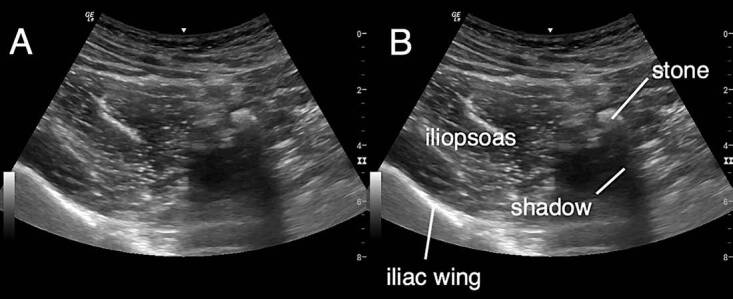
Ultrasound right lower quadrant (RLQ), short axis, curvilinear probe The landmarks of a RLQ ultrasound can be noted in short axis using the curvilinear probe with the iliac wing and iliopsoas muscle in view. Medial to the iliopsoas a hyperechoic focus representing a stone with acoustic shadowing is noted.
